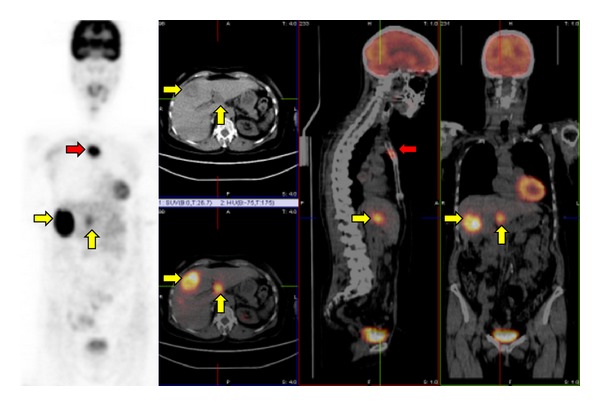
FDG-PET/CT in a 68-year-old female previously operated for HCTC showing the presence of sternal (red arrows) and liver metastases (yellow arrows).
